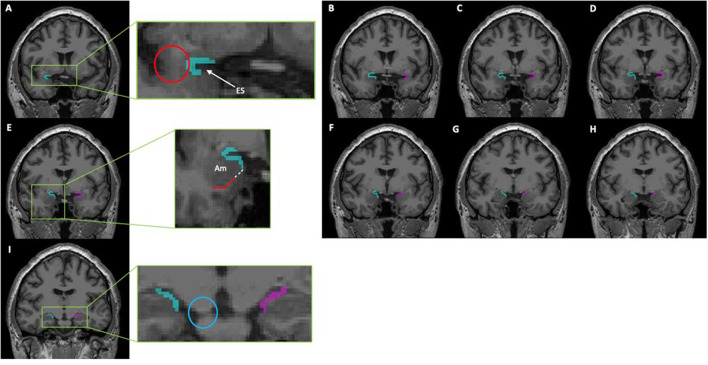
This series of coronal T1-weighted MR-images depicts a segmentation of the piriform cortex using ITK-SNAP (Version 3.8.0). From rostral to caudal, the piriform cortex can be identified as follows: (A,B) The most rostral portion of the piriform cortex is denoted by the presence of the limen insulae (Red Circle); here it will consist of both a frontal- and temporal-component that creates a “C” shape around the entorhinal sulcus (ES). (C–E) The temporal portion of the piriform cortex is progressively extended until reaching the gyrus semiannularis. If the gyrus semiannularis was not easily identified, then a parallel line (white dotted line) extending from the white matter (red solid line) under the amygdala (Am) was made toward the surface of the cortex, and the temporal piriform was extended to that point. (F–H) As the optic tracts move laterally and merges with the temporal cortex, the frontal component of the piriform cortex recedes correspondingly. (I) Only the temporal piriform cortex can be seen; the most caudal portion of the piriform cortex is seen on the the coronal slice just before the cerebellar peduncle completely merges with the pons (Blue Circle). This protocol was adapted from those previously described by Goncalves Pereira et al. (7), Galovic et al. (5), and Iqbal et al. (10).
